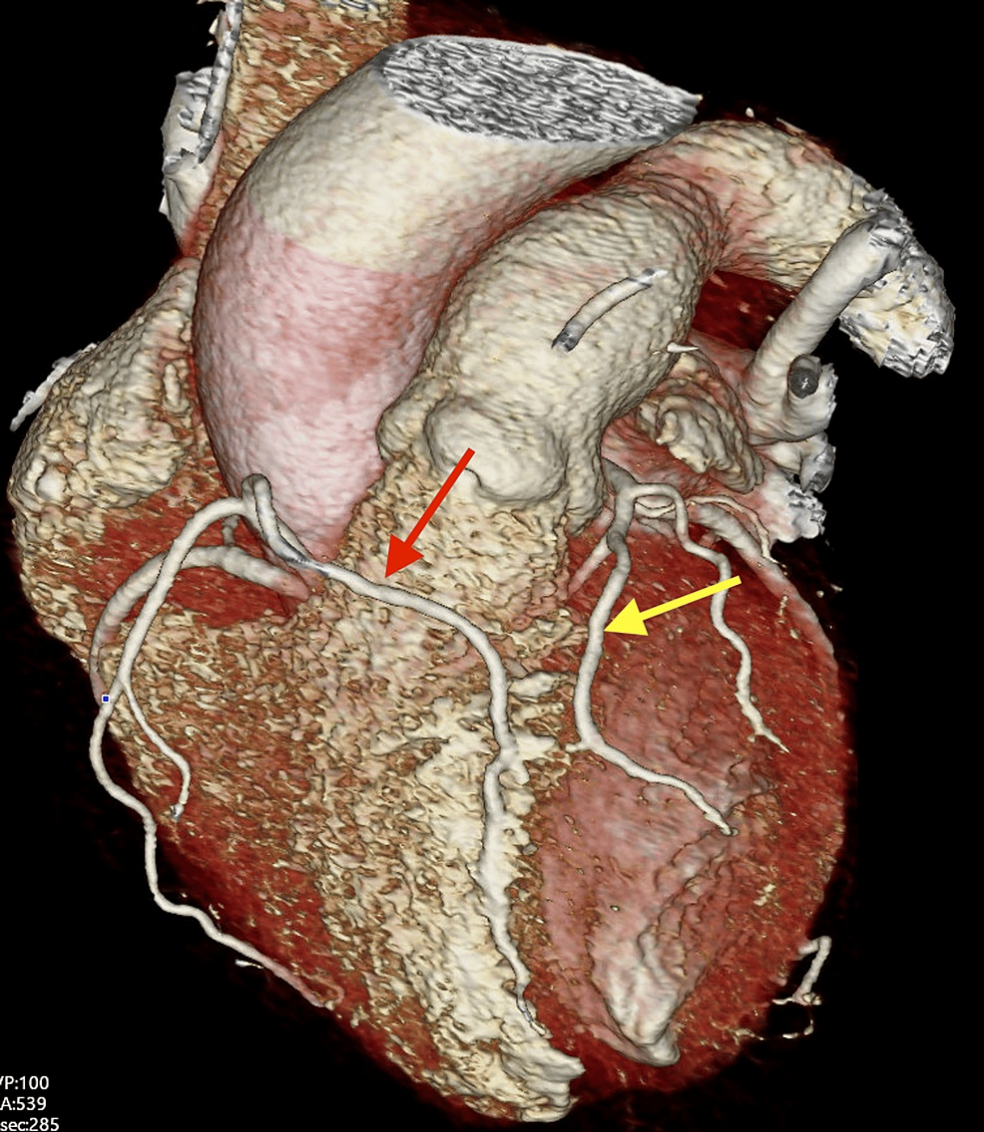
Three-dimensional volume-rendered cardiac computed tomography (CT) depicting long LAD (red arrow) arising from the right sinus of Valsalva and eventually traveling into the anterior interventricular groove. Additionally, the short LAD (yellow arrow) is shown to arise from the left sinus of Valsalva and course along the interventricular septum. LAD: left anterior descending
